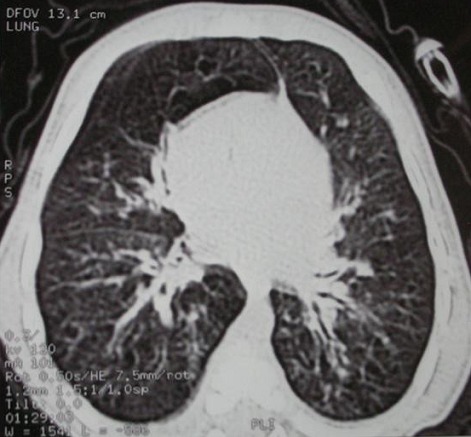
Chest CT scan showing bronchiectasis in a patient suffering from cystic fibrosis
